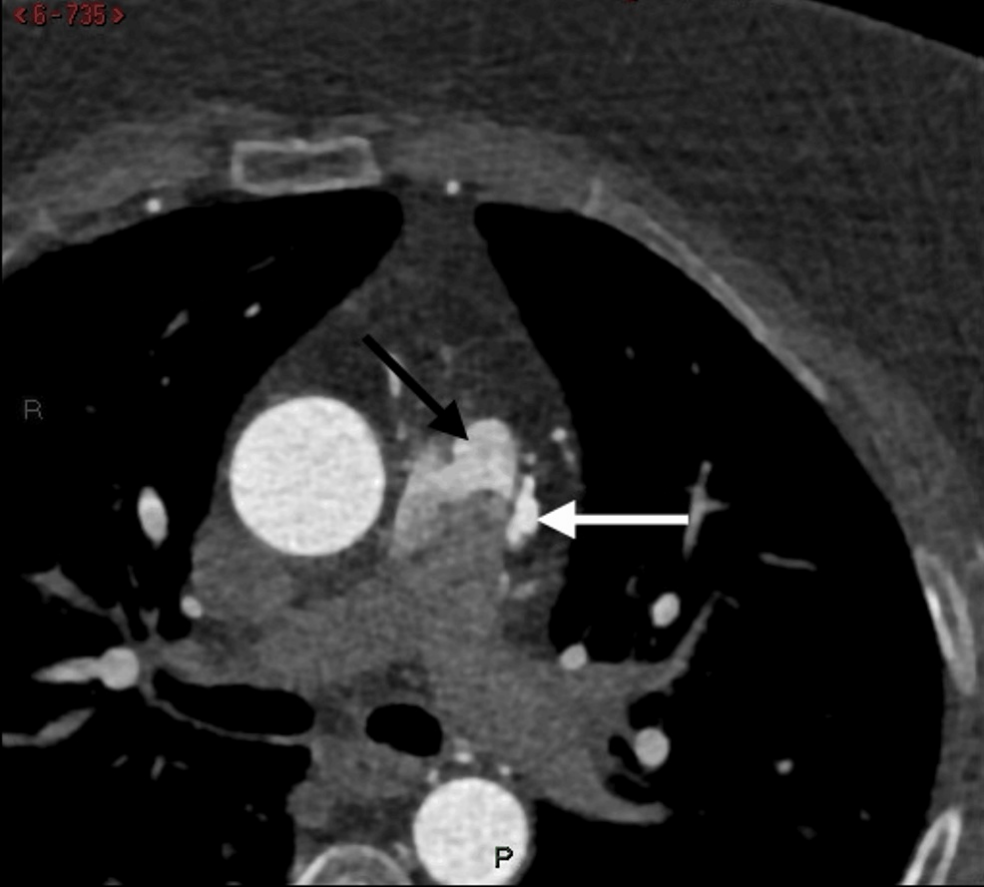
An axial CT image shows a typical pierced sign and the smoke sign, resulting from a jet of contrast flushing into the main pulmonary artery (black arrow) and small focal aneurysm formation (white arrow).
